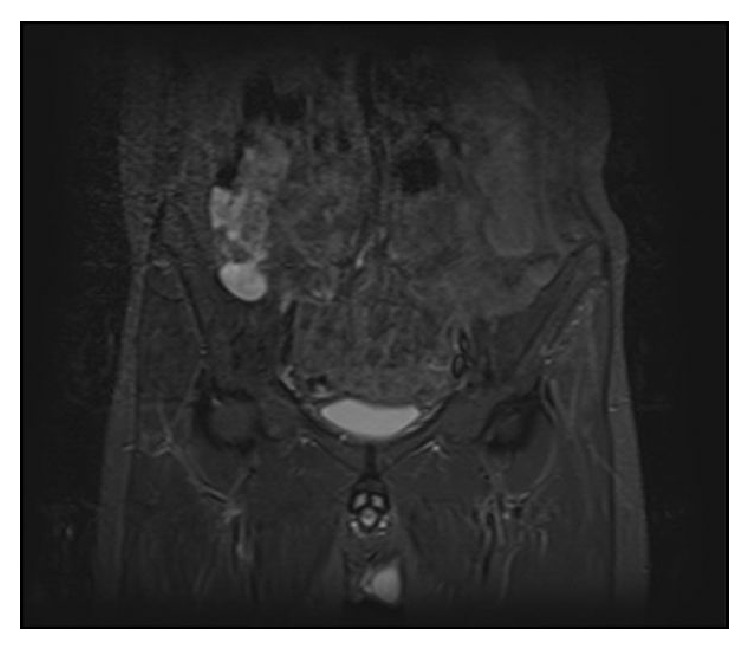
MRI of accumulated suppurative fluid within the iliopsoas fascia.
